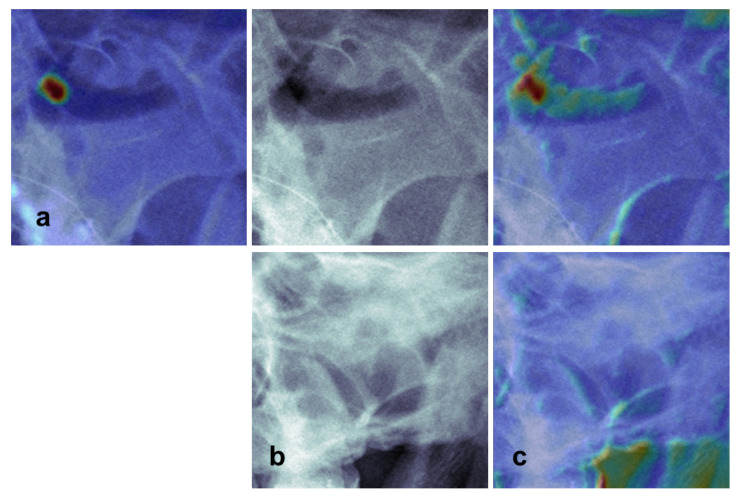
Comparison of the class activation maps (CAMs) of the single primary view and multi-view model. (a) CAM of the single primary view model (Waters’ view). (b) Original radiographs showing maxillary sinusitis with air-fluid level (upper row, Waters’ view; lower row, Caldwell view) (c), CAM of the multi-view model (upper row, Waters’ view; lower row, Caldwell view). Single primary view model (a) misclassified as mucosal thickening because the area above the horizontal fluid line is not recognized. In the case of the multi-view model (c), the prediction is correct as the activated area is more expanded on Waters’ view (upper row), while no clear activation is found in the sinus area on the secondary view (lower row).
